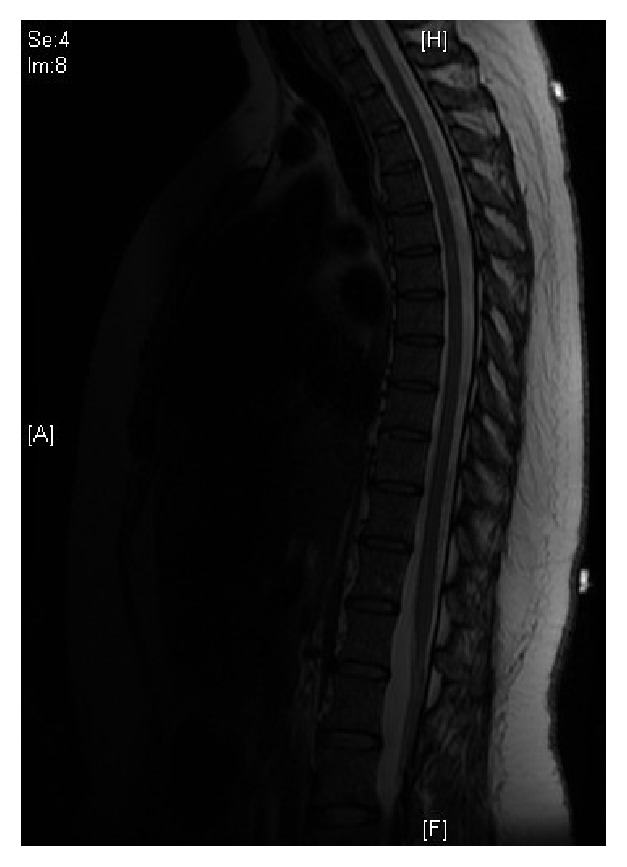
MRI at 6 weeks postpartum showing complete resolution of the spontaneous epidural hematoma.
